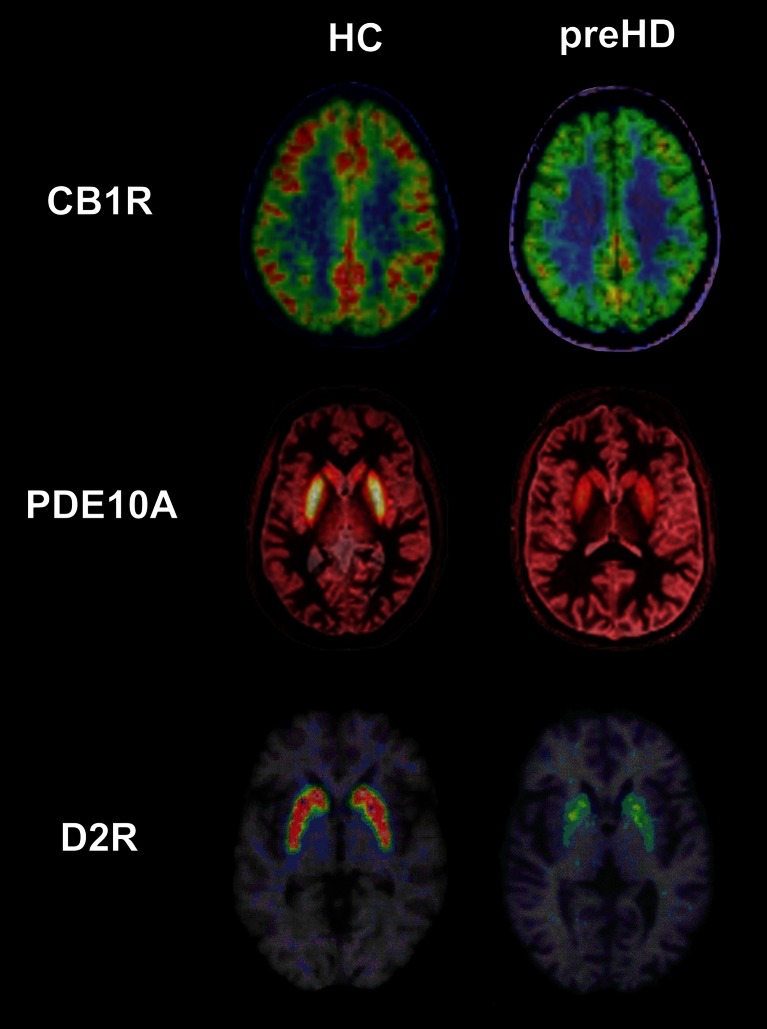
Example PET images in healthy controls (HC) and premanifest Huntington’s disease gene carriers (preHD). Axial [^11^C]MePPeP, [^11^C]IMA107, [^11^C]raclopride images in stereotaxic space overlaid onto the T1-weighted MNI template show decreased cortical CB_1_ receptor, striatal PDE10A and striatal D2 receptor binding in the preHD subjects compared to the healthy controls . Images from our data
